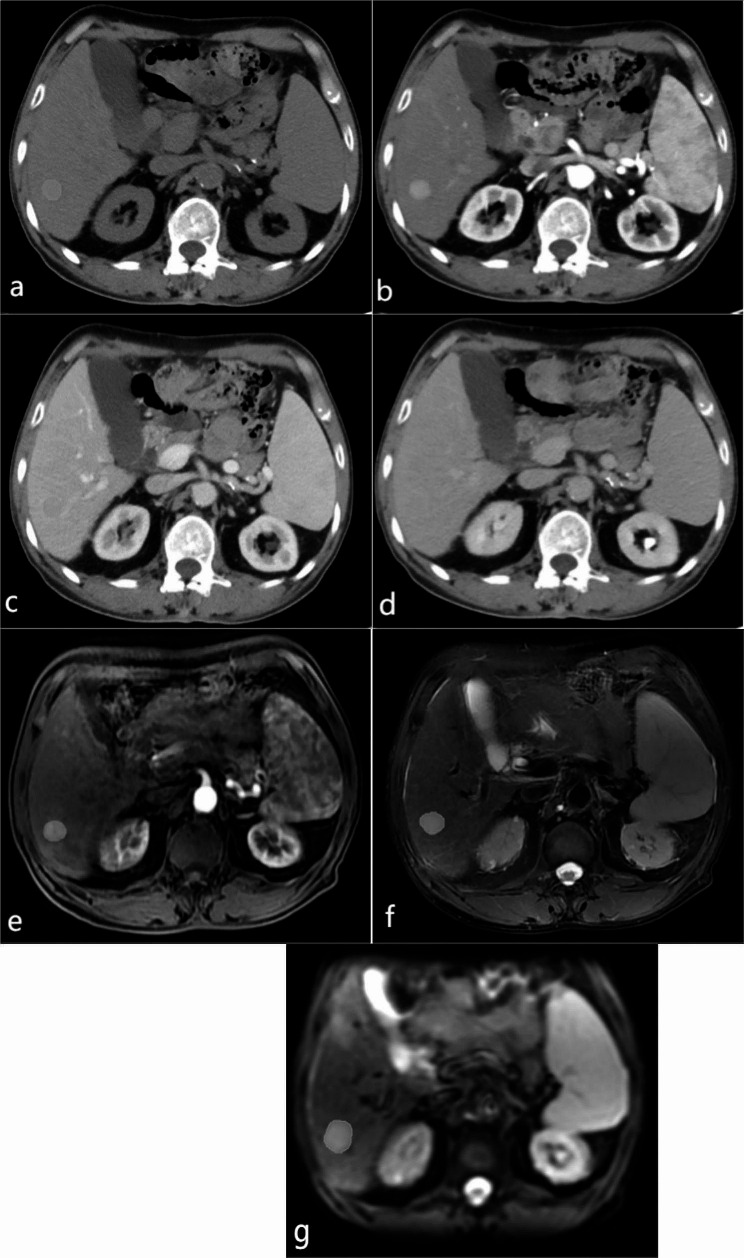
A 62-year-old male patient with HCC. A-D show CT axial images: A plain scanning phase, B arterial phase, C portal venous phase, D delayed phase; E-G show MRI axial images: E arterial phase, F T2WI, G DWI
